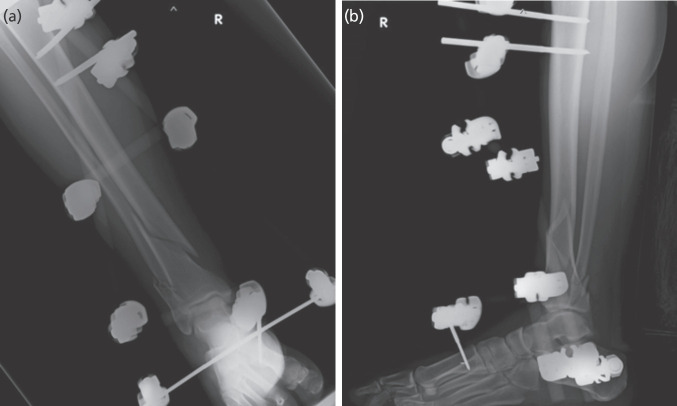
Radiographs of the ankle joint after stabilisation with external fixator. (a) Antero-posterior view. (b) Lateral view.
